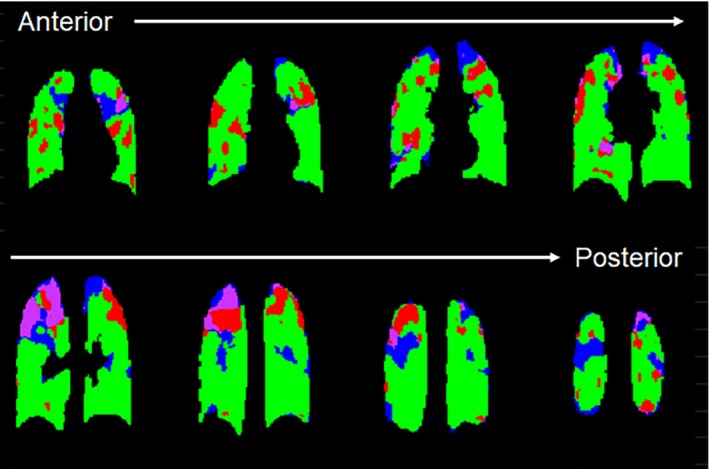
The overlap of the ^129^Xe‐MRI (Xe‐MRI) and 3D phase‐resolved functional lung (PREFUL) MRI derived ventilation defects are shown for all lung slices (anterior/posterior) in a representative pediatric CF patient, with 3D VDP_RVent_ = 22.9% and VDP_Xe_ = 19.2% (purple regions = both 3D VDP_RVent_ and VDP_Xe_ red regions = only 3D VDP_RVent_ blue regions = only VDP_Xe_ and green regions = neither VDP_Xe_ nor 3D VDP_RVent_). The Dice similarity coefficient (DSC) values of the healthy and defect regions are 0.75 and 0.24, respectively. CF, cystic fibrosis; VDP, ventilation defect percentage.
